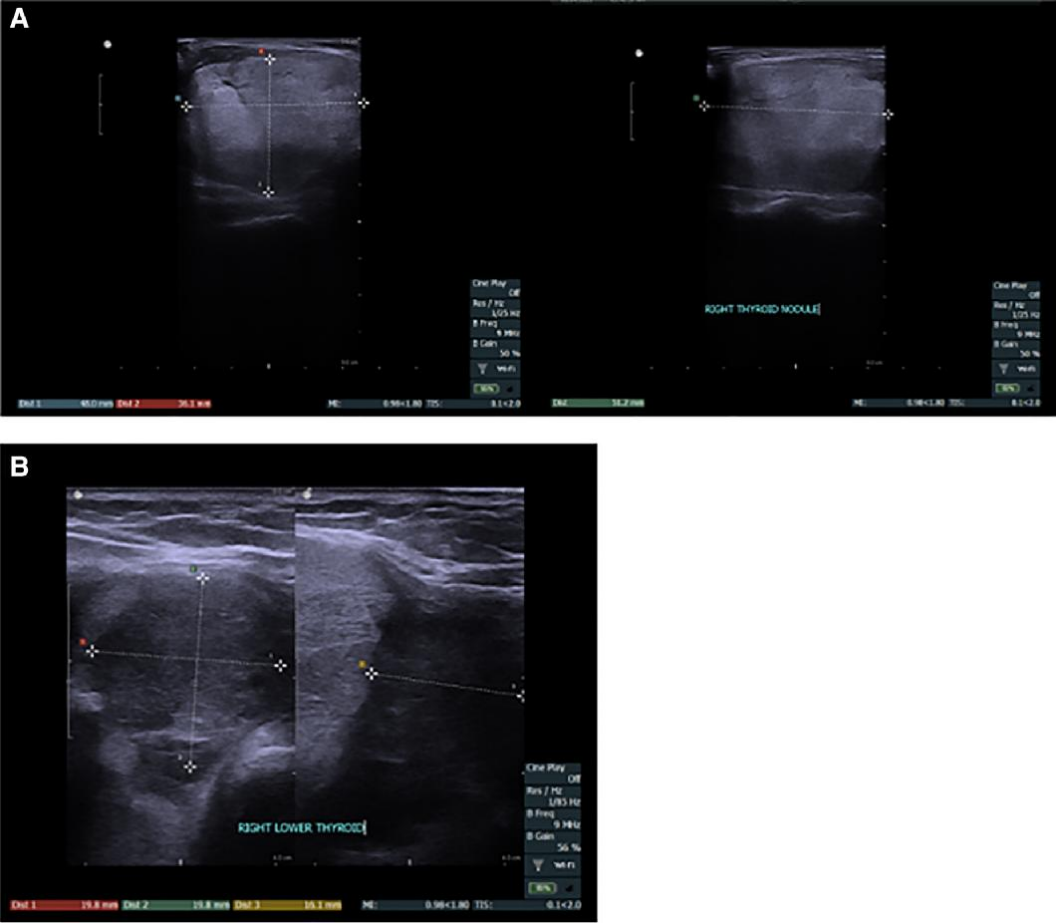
Thyroid ultrasound. A, Right superior-middle pole solid isoechoic thyroid nodule (4.8 × 3.6 × 5.1 cm). B, Right lower solid hypoechoic thyroid nodule (2.0 × 2.0 × 1.6 cm).
